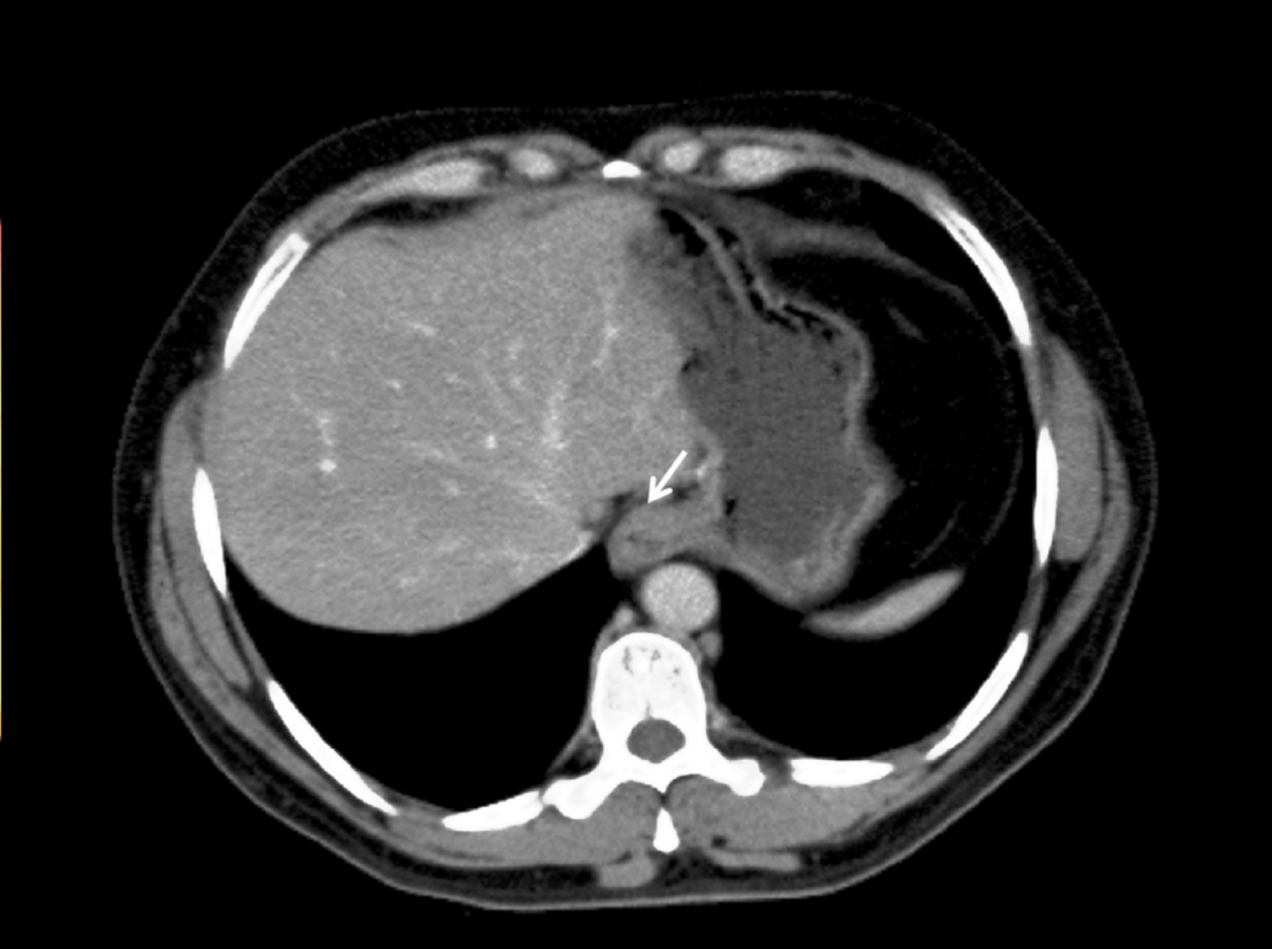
Contrast-enhanced computed tomography axial section (soft tissue window) at the level of diaphragm showing herniation of the part of the stomach through the esophageal hiatus into the thoracic cavity (arrow).
